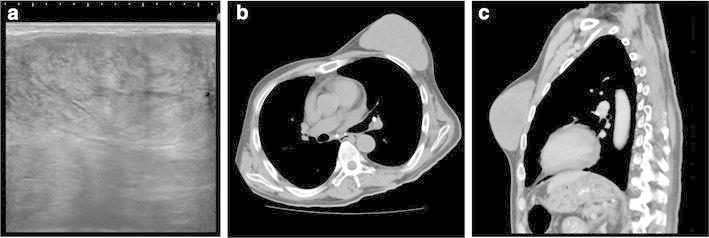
Imaging examination shows a tumor in the left breast. Ultrasonography shows an oval and low-echo tumor, with a heterogeneous internal echo, that possessed slit-like spaces (a). Computed tomography shows a well-defined lobular tumor with a maximum diameter of 11 cm in the left breast. b Transaxial section; c sagittal section.
